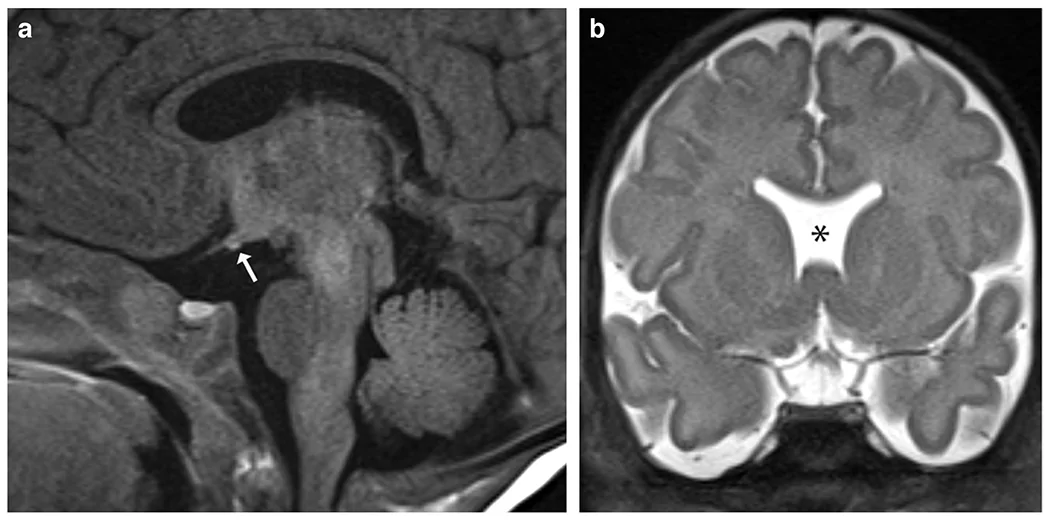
Twelve-day-old male infant with a prenatal diagnosis of absence of the septum pellucidum and postnatal diagnoses of bilateral optic nerve hypoplasia, central hypothyroidism and partial adrenal insufficiency. a Sagittal T1-weighted midline image focused on the sella shows an ectopic neurohypophysis positioned at the median eminence of the hypothalamus (*arrow*). b Coronal T2-weighted brain magnetic resonance image shows absence of the septum pellucidum (*asterisk*)
